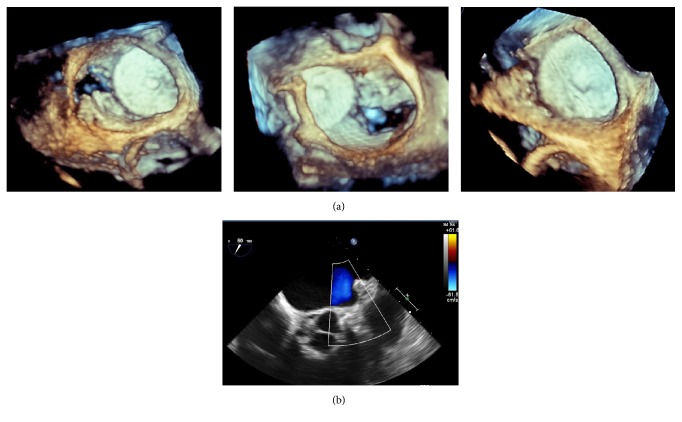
Using 3D transesophageal echocardiography, (a) a stable position of the device within follow-up period could be confirmed (*from left to right*: immediately after implantation, after 3 months and 6 months). Color Doppler TEE revealed complete device occlusion of the LAA (b).
